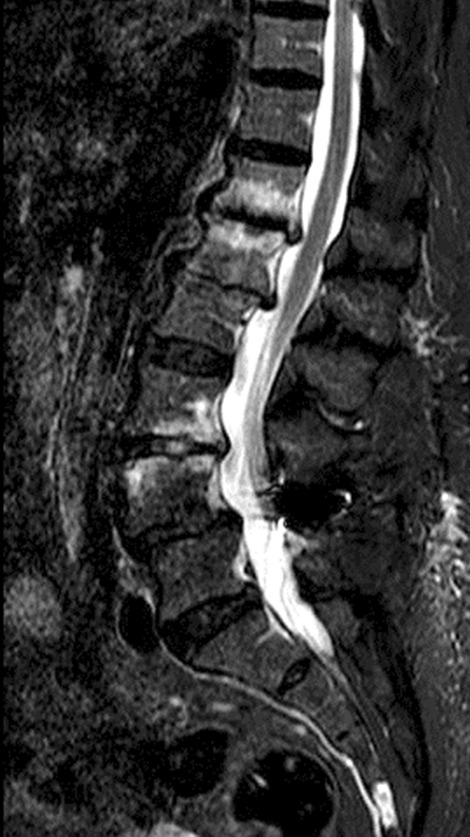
Sagittal stir magnetic resonance imaging
